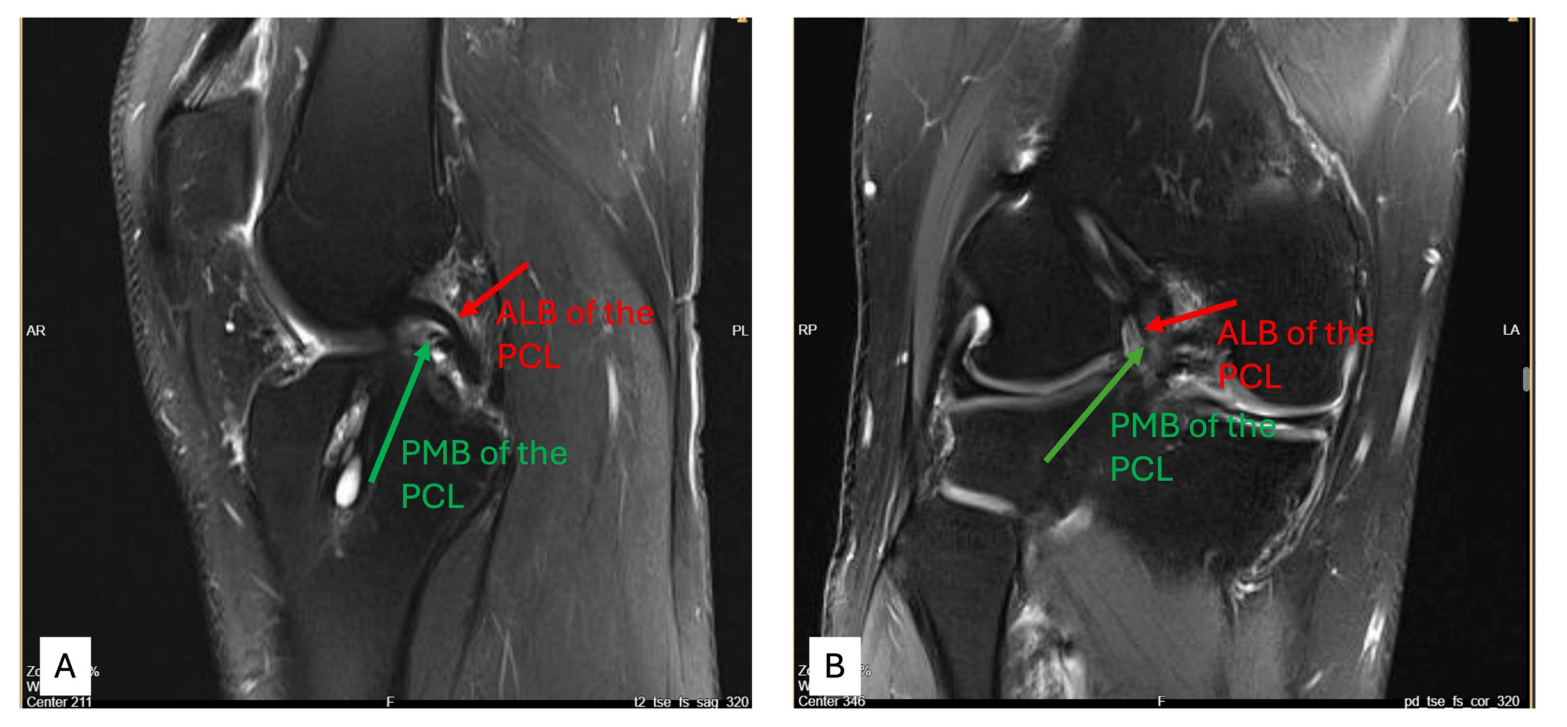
Preoperative MRI demonstrating rupture of the PMB of the PCL (A) Sagittal T2-weighted image showing disrupted and irregular PMB signal. (B) Coronal T2-weighted image confirming loss of continuity in the PMB. MRI: magnetic resonance imaging, PMB: isolated posteromedial bundle, PCL: posterior cruciate ligament, ALB: anterolateral bundle
